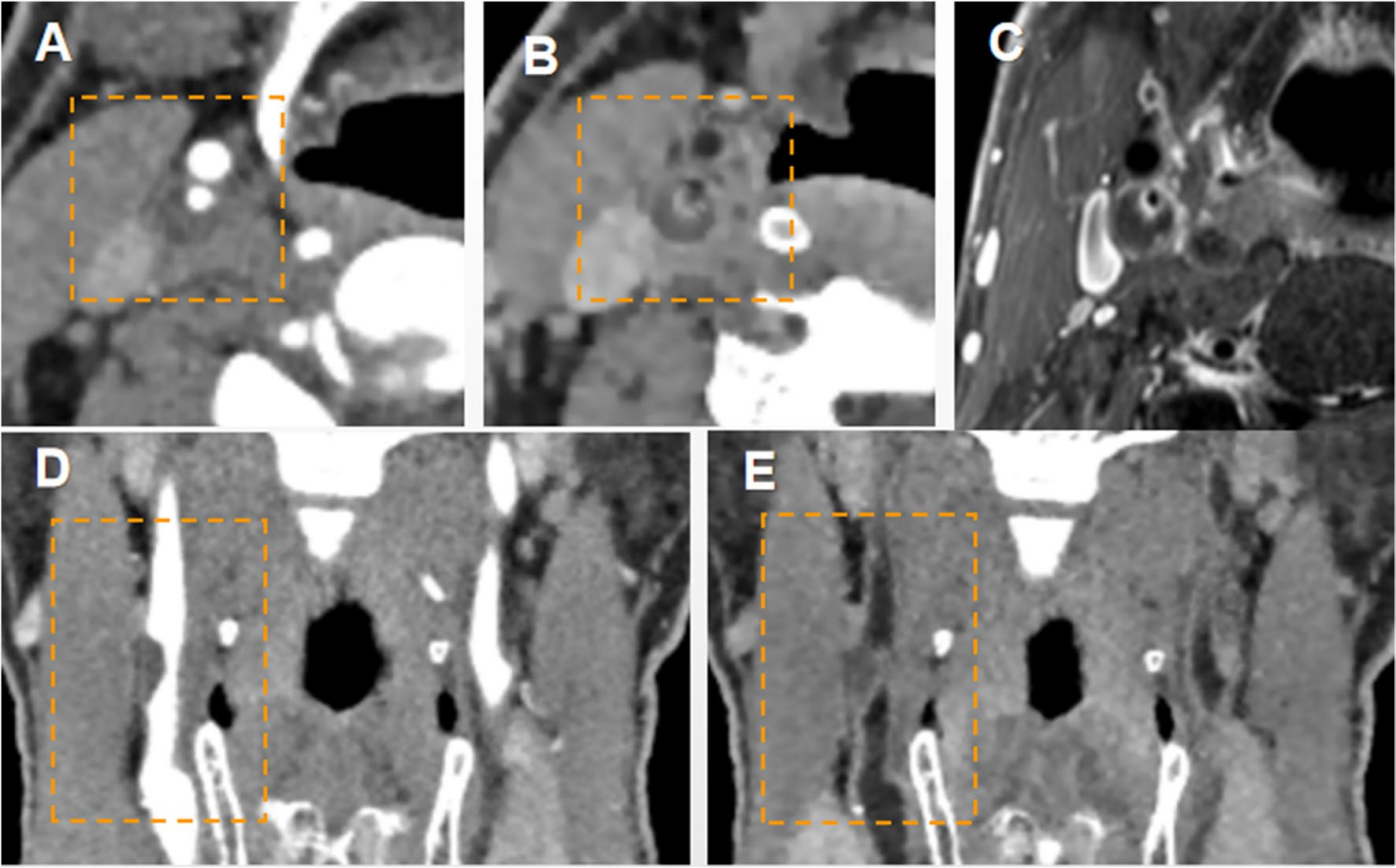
A 65-year-old man with a hypodense plaque in the right internal carotid artery which induced severe luminal stenosis. On the axial images, BBCT (B) was better than CTA (A) in displaying low-density plaques. VW-MR images (C) showed an eccentric vulnerable plaque; D, E Multi-planar reformatting (MPR) coronal view of CTA and BBCT, respectively
